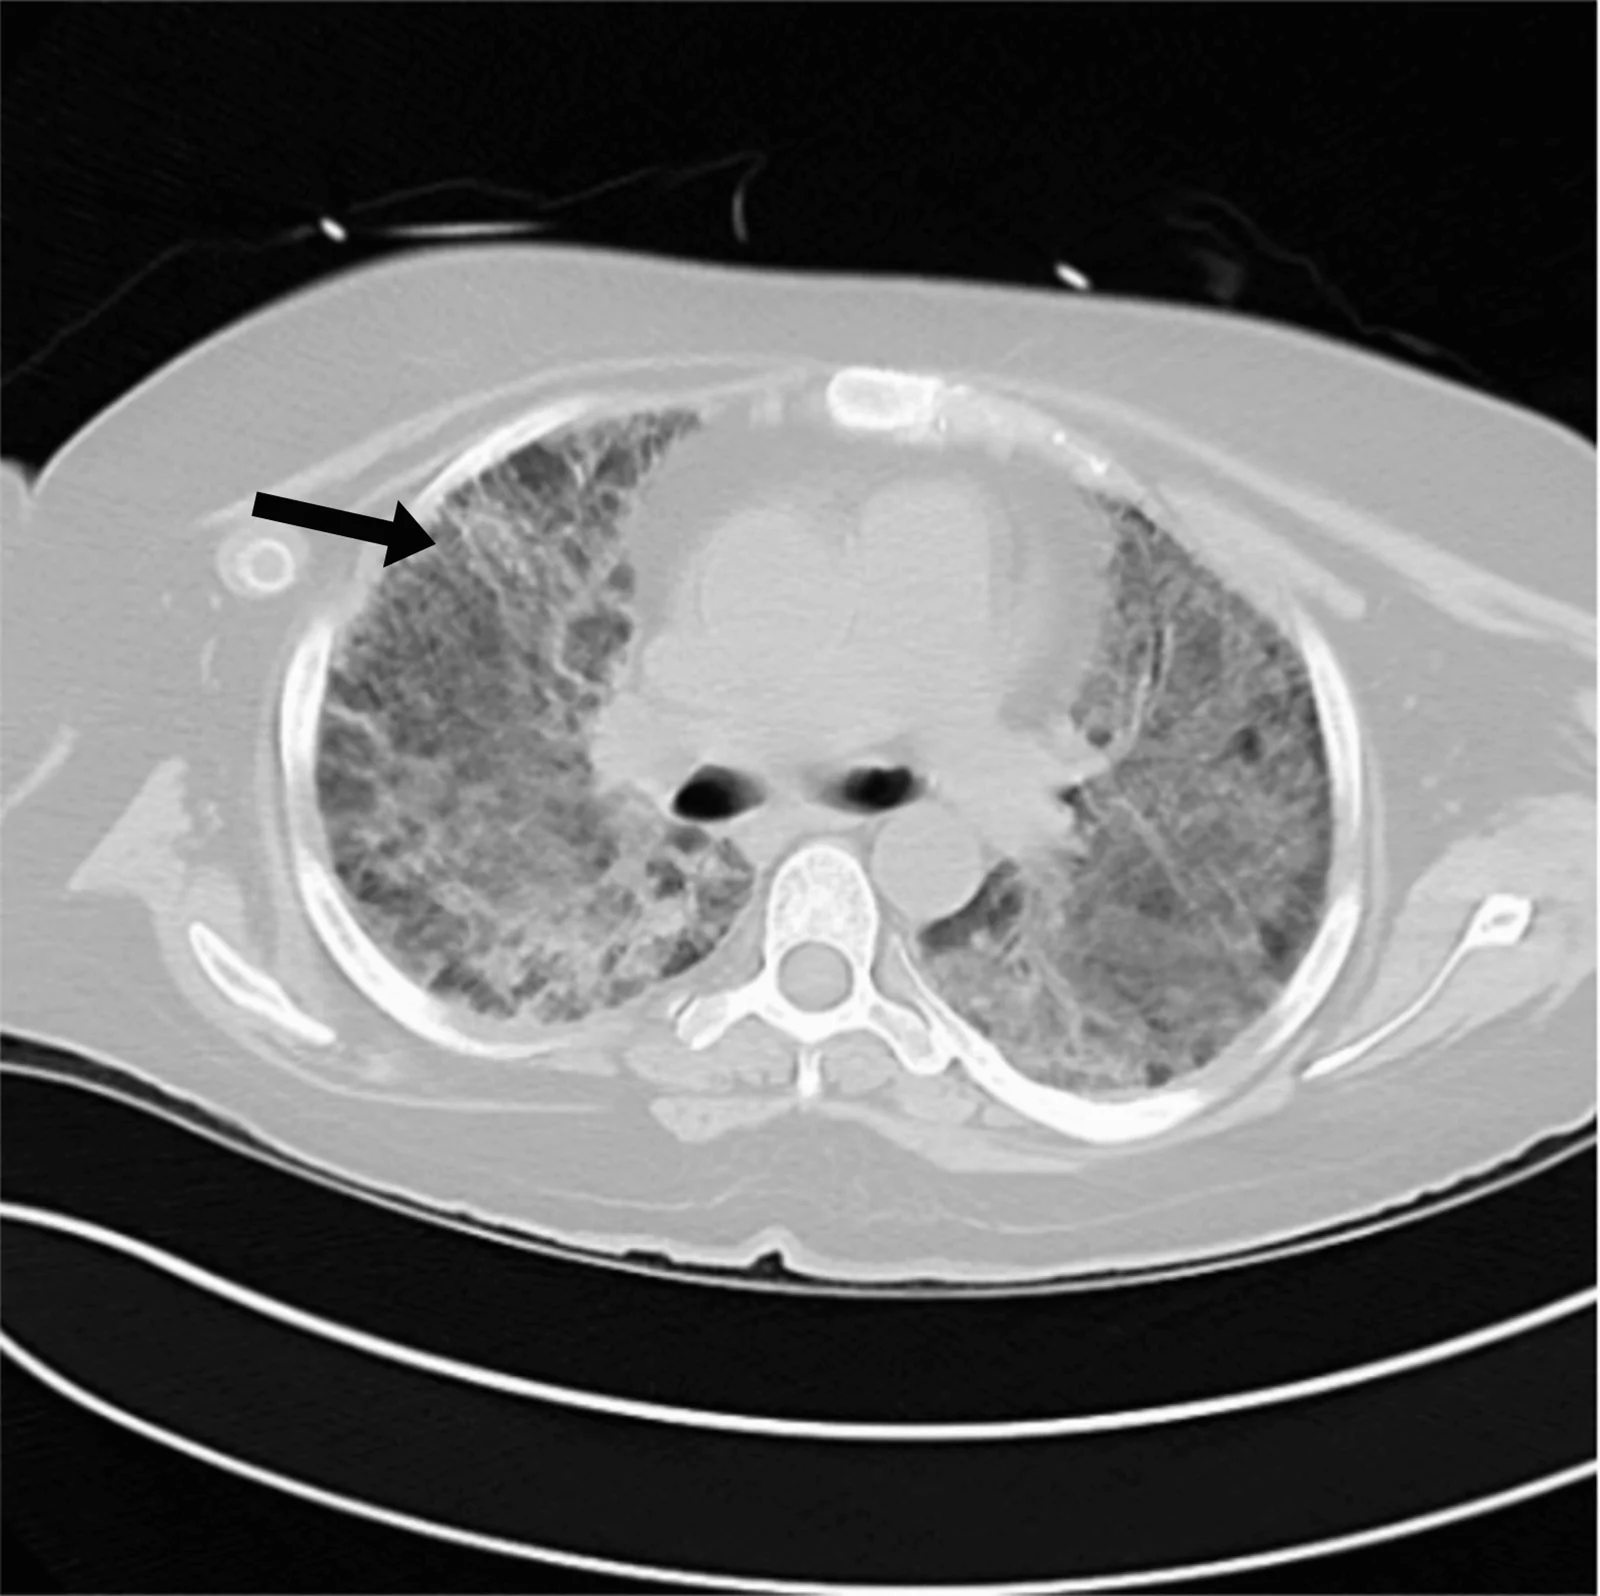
Non-contrast chest CT obtained eight days into readmission, demonstrating persistent, diffuse ground glass opacities (black arrow).
